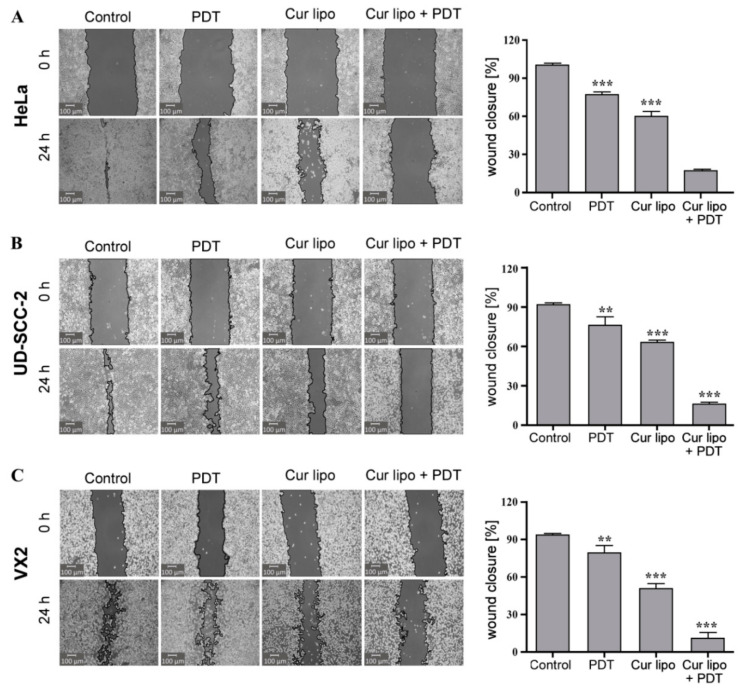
Cell migration (wound healing) assay in HeLa, UD-SCC-2, and VX2 cells. HeLa (A), UD-SCC-2 (B), and VX2 (C) tumor cells were treated with photodynamic therapy (PDT) only, curcumin liposomes (Cur lipo) only, or Cur lipo in combination with PDT. Images were captured at *t* = 0 h directly after scratching the cell layer and *t* = 24 h to evaluate scratch (wound) closure indicative of cellular migration. The cell-free area of the scratched region was measured with the Montpellier Ressources Imagerie (MRI) wound healing tool used with the ImageJ analysis software [41]. The level of cell migration is presented as the percentage of scratch (wound) closure observed 24 h after treatment compared to control values. Controls indicate untreated cells. The values are expressed as the mean ± standard deviation, and statistical significances are indicated as * *p* < 0.001,  *p* < 0.01.
